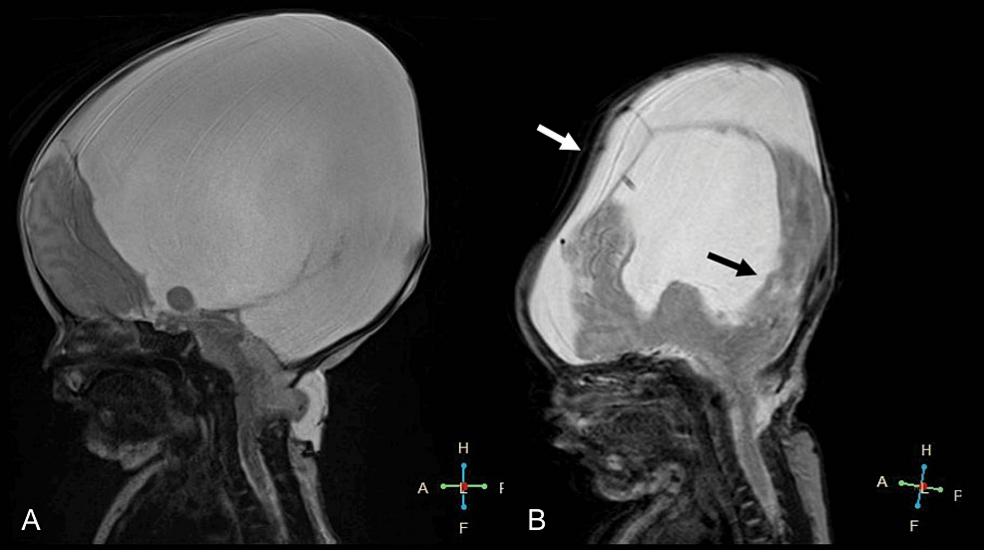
Comparison of pre- and post-procedural MRI T2 sagittal images of the head A) Pre-procedural MRI; B) Post-procedural MRI, the black arrow highlights the recovery of brain volume and the white arrow points to the improvement in hydrocephalus MRI, magnetic resonance imaging
